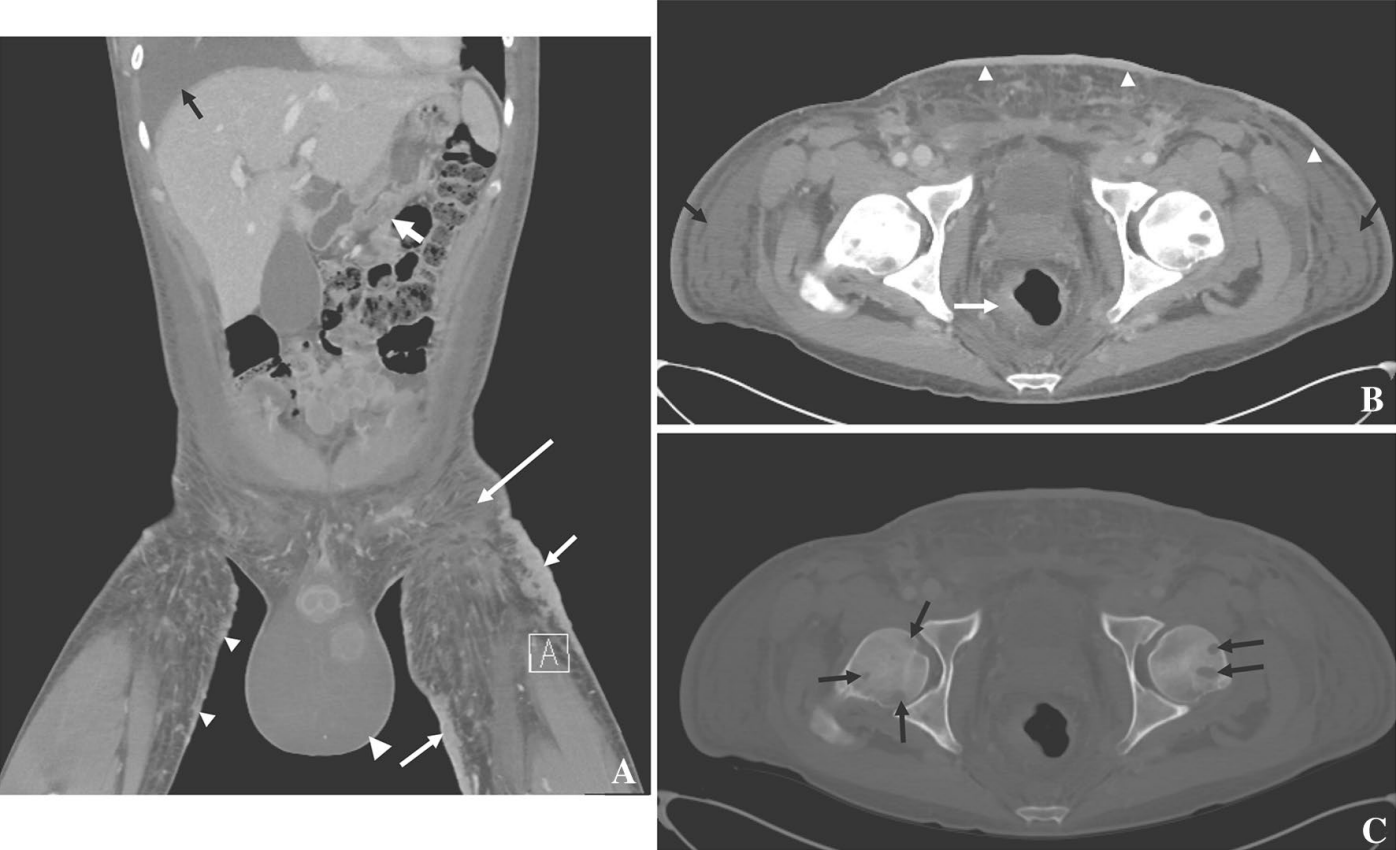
33-year-old male with HIV and KS. A Coronal contrast-enhanced CT showing antral wall thickening (fat white arrow), large and thick skin thickening (plaques, short white arrows), small skin nodules (small arrowheads), hydroceles (large arrowhead), subcutaneous edema (long arrow), and a pleural effusion (black arrow). B Axial contrast-enhanced CT showing asymmetric rectal wall thickening (white arrow), skin thickening (arrowheads), and subcutaneous edema (black arrows); C bone windows show lytic bone metastases
